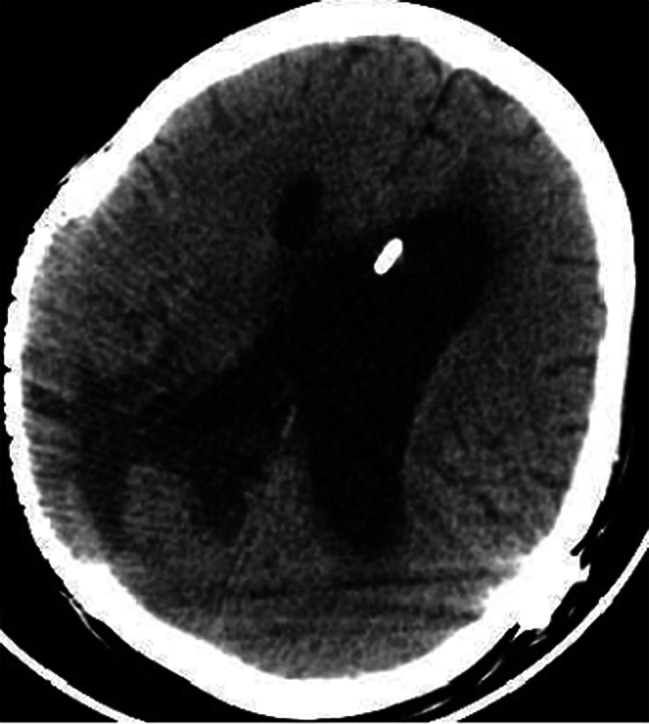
Post-operative changes were observed in the right skull and left frontal bone. Patchy low-density shadows were observed in the right parietal–occipital lobe with unclear boundaries (axial cut of CT scan of the brain)
